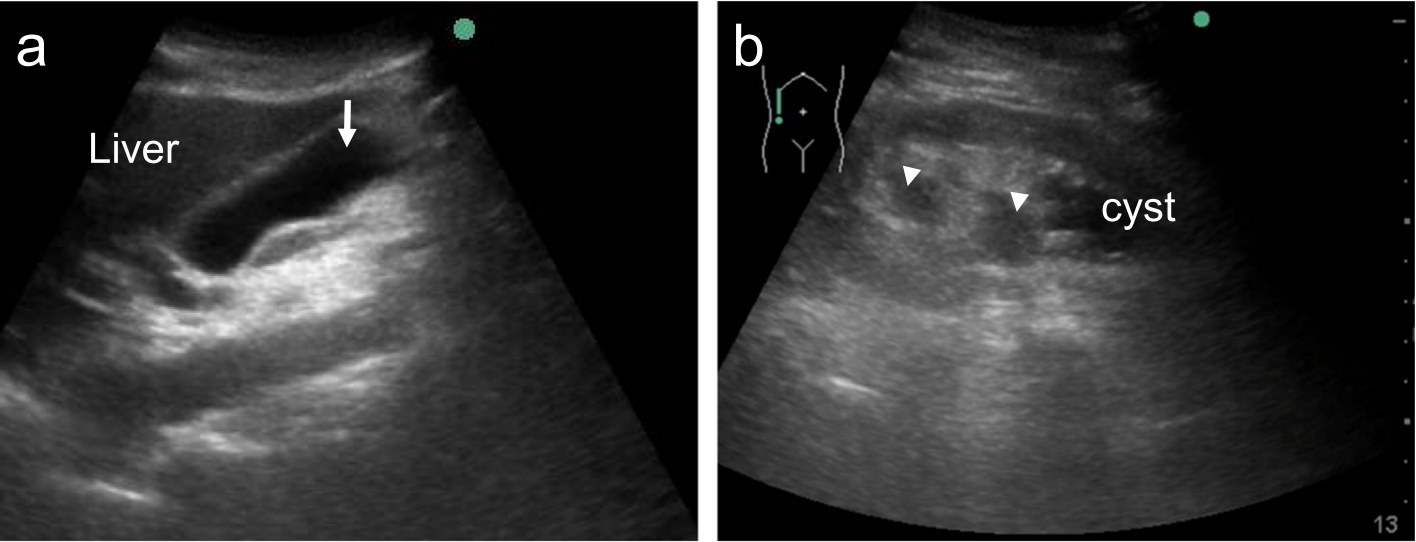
Ultrasound images in an 88-year-old man who presented with shaking chills. The patient had a history of acute cholecystitis with percutaneous transhepatic gallbladder drainage. On physical examination, he had no abdominal or costovertebral angel tenderness. Bedside ultrasound showed a normal gallbladder (a, *arrow*) and pelvic dilatation in the right kidney (b, *arrowheads*). A subsequent computed tomography scan revealed the stone at the right ureterovesical junction. A complicated urinary tract infection was strongly suspected, and emergent urological consultation was ordered. He fell into shock soon after the initial evaluation
